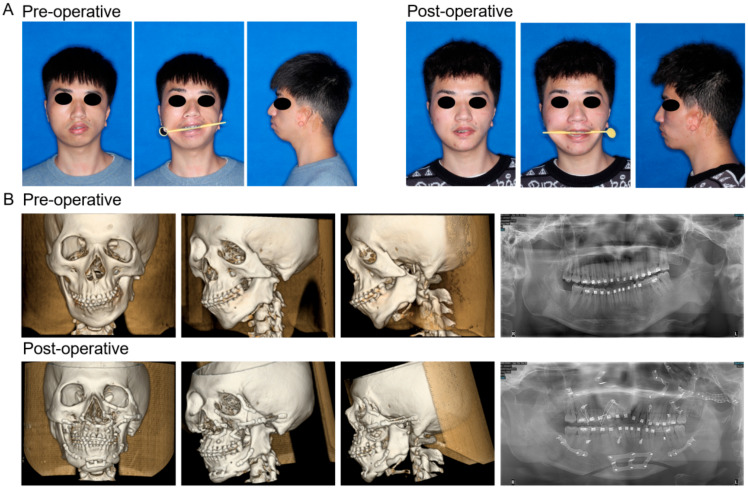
Preoperative and postoperative (6 months later) facial photographs (A), CT images, and panoramic radiographs (B).
